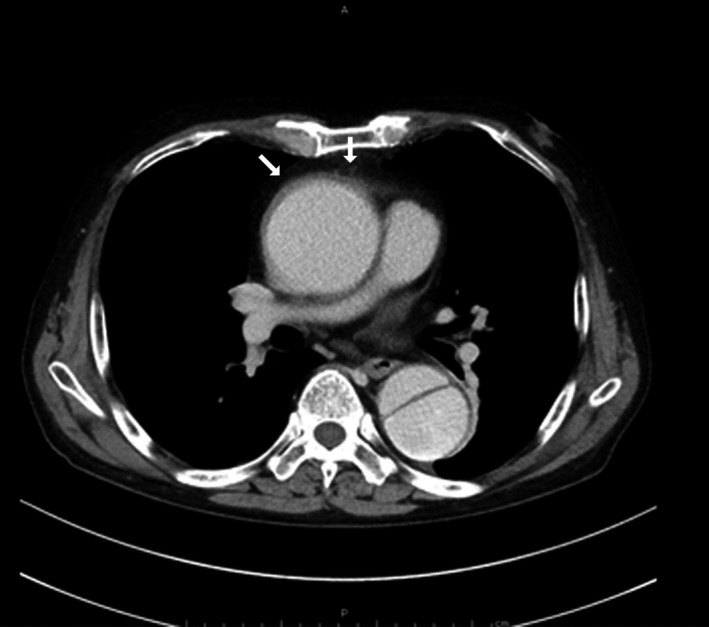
Contrast‐enhanced chest computed tomography. A dilatation of the ascending aorta and a dissection of the descending aorta are observed. The soft tissue around the ascending aorta is thickened showing irregular surfaces (arrows).
